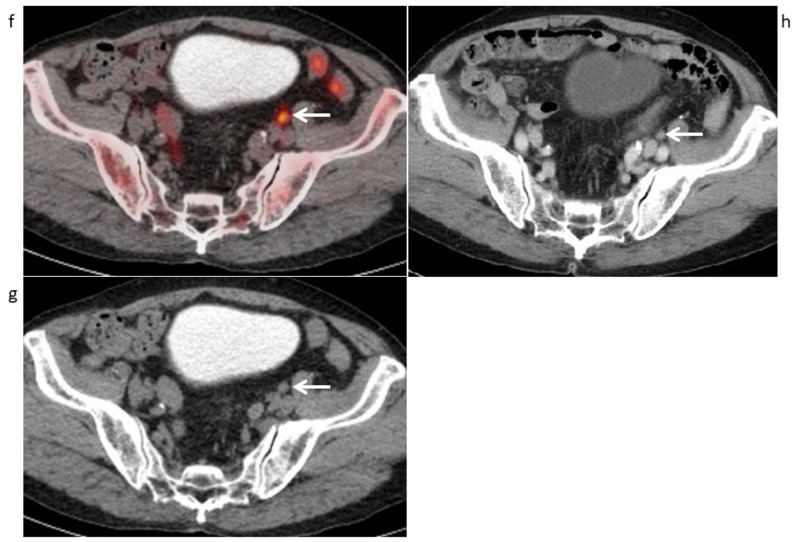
Axial 11C-choline PET/CT and contrast-enhanced CT (Case 1) (f) Axial 11C-choline PET/CT and (g) CT part show mild swelling measuring 7 mm in short diameter and abnormal 11C-choline uptake (SUVmax 3.42) of the left external iliac node (arrow), with a score of 5 for diagnosing lymph node metastasis. (h) Contrast-enhanced CT shows mild swelling of the left external iliac node measuring 7 mm in short diameter (arrow), with a score of 3 for diagnosing lymph node metastasis.
